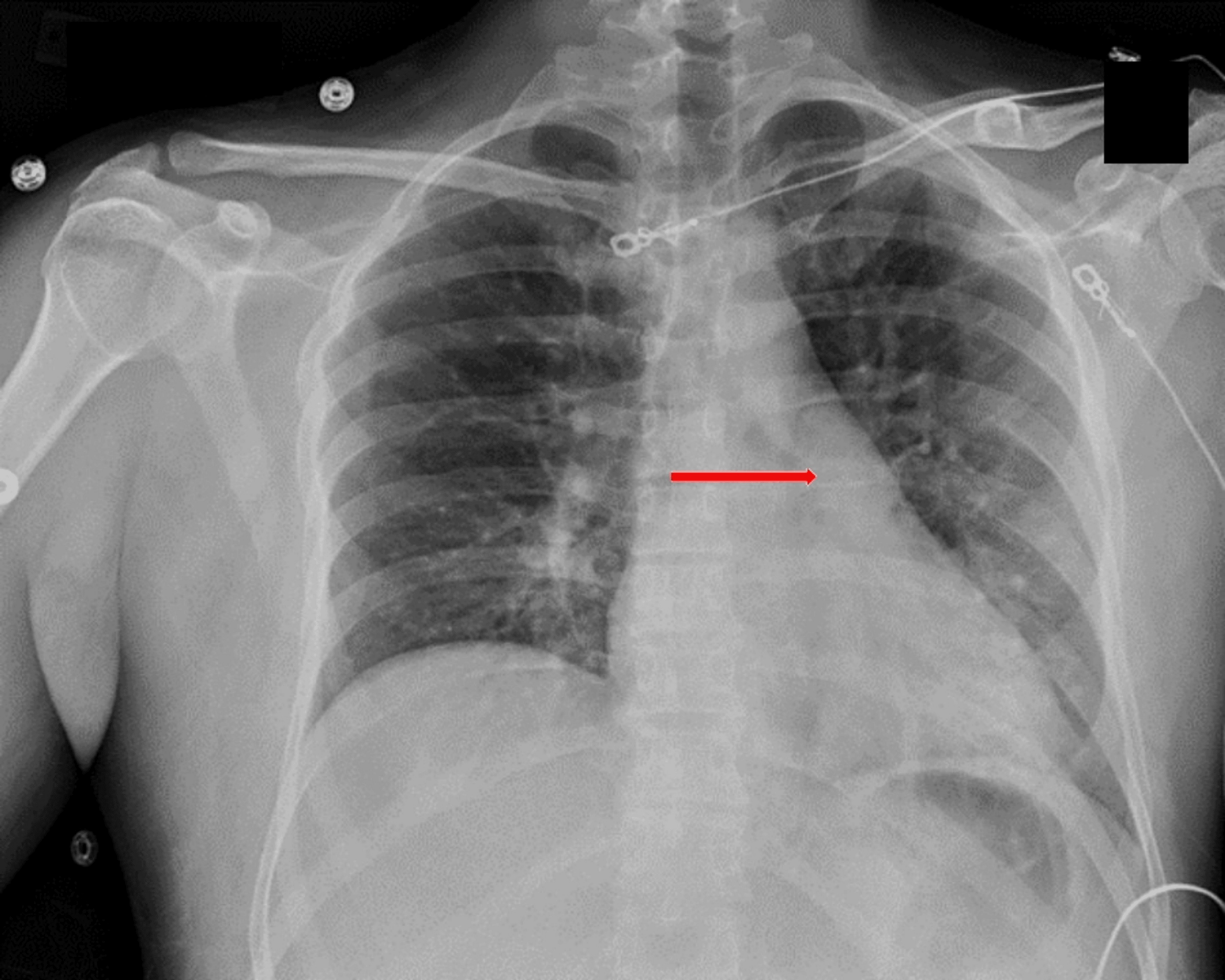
Chest radiograph with arrow showing opacification in the left lower lobe
